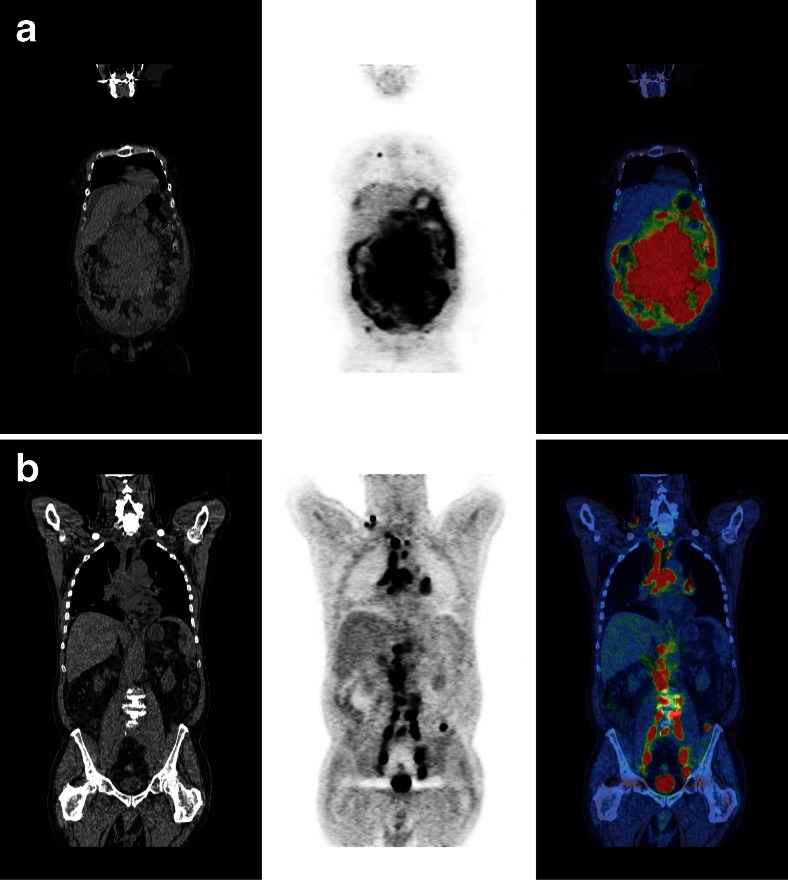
Coronal CT, PET and fused images are shown of patients with high metabolic tumour volume at baseline which is predictive of inferior prognosis. The patient in the *top panel* (a) had bulk disease by conventional assessment of maximal tumour dimension; the patient in the *bottom panel* (b) does not have bulky disease
